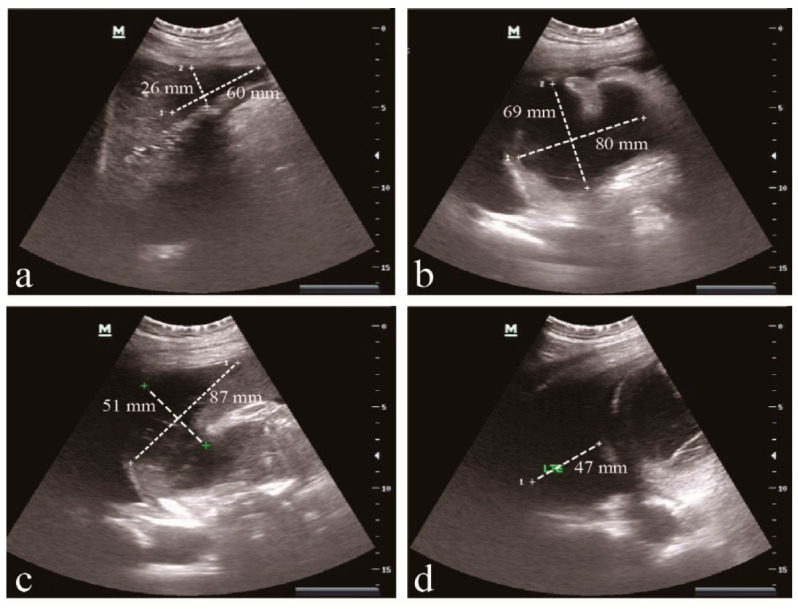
Abdominal ultrasonography. (a) The hepatic abscess (60 × 26 mm) was located in the liver’s left lobe. (b) Spleen fossa abscess (80 × 69 mm). (c) Anechoic area near the spleen (87 × 51 mm). (d) Pleural effusion on the left (47 mm).
